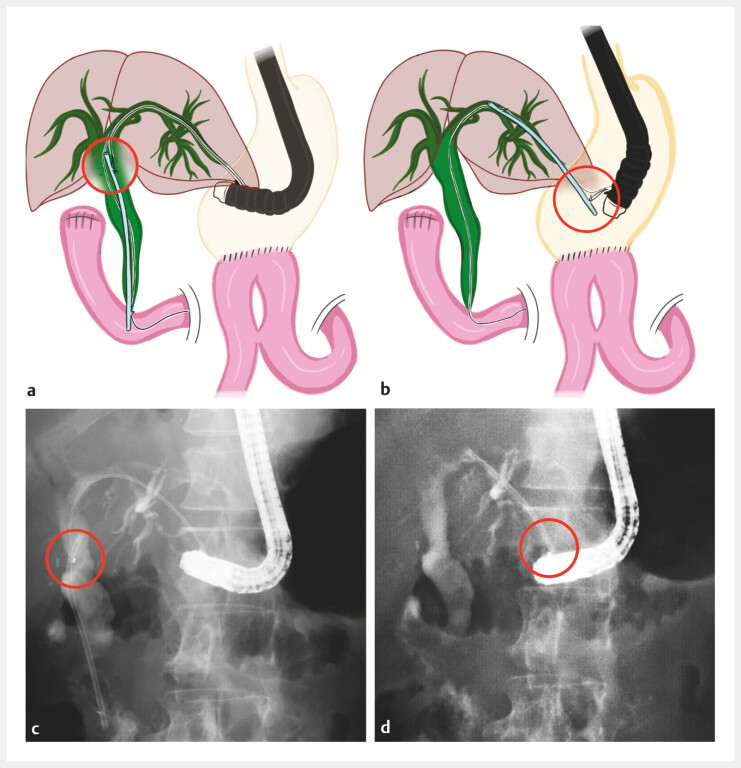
Repositioning a plastic stent via the endoscopic ultrasound-guided hepaticogastrostomy or hepaticojejunostomy (HGS/HJS) route using the handmade snare. In all panels, the grasping portion of the plastic stent is indicated by red circles.
